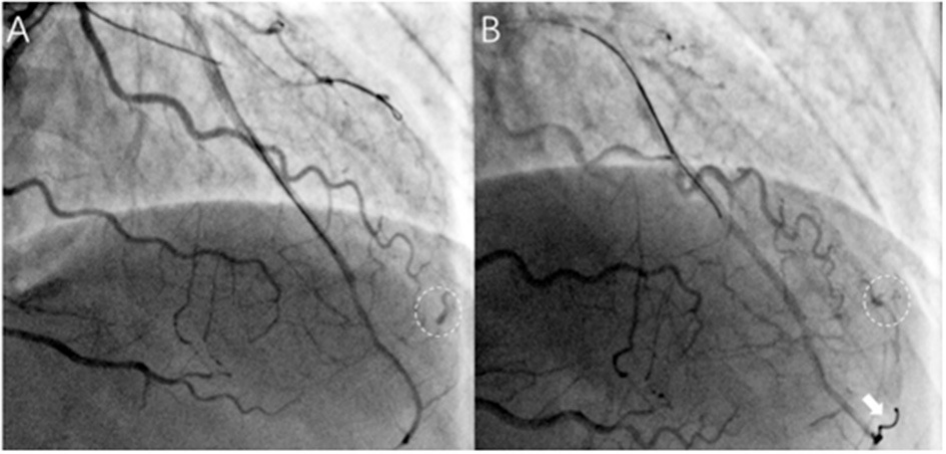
Acquired coronary fistulas uselessly and unnecessarily treated with coil embolization in a patient. (A) After successful recanalization of the LAD, ACFs were visualized and difficult to identify with coronary perforation timely (circle); (B) Rattled and successful delivery of one coil through the microcatheter (Finecross, Terumo, Japan) (arrows), but ACFs still existed (as shown in circle), and pericardial effusion was not found on echocardiography. (Supplementary Movies 9, 10 show angiographic findings).
